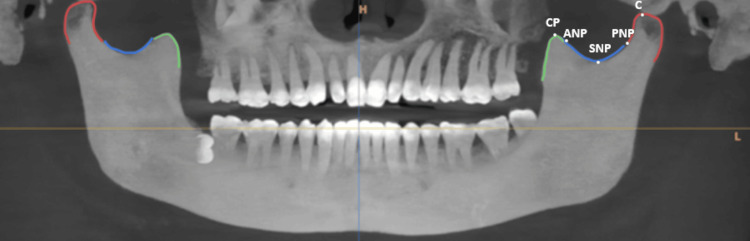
Tracing of the coronoid process, condyle, and sigmoid notch in a CBCT image CBCT: cone beam computed tomography; CP: coronoid process; ANP: anterior notch point; SNP: superior notch point; PNP: posterior notch point; C: condyle
